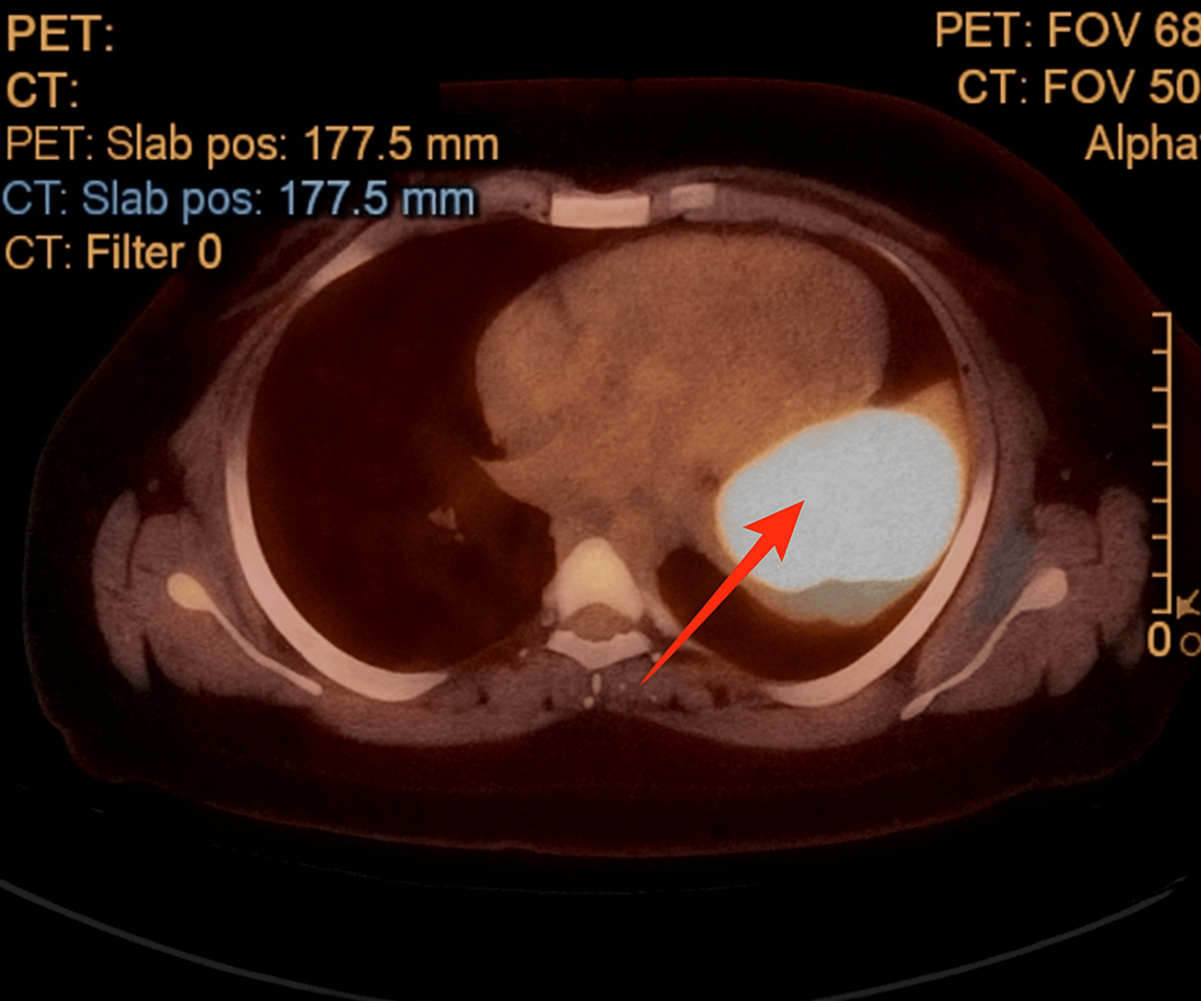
PET/CT scan image performed in April 2023 The image shows a hypermetabolic left upper lobe mass (red arrow). PET/CT: Positron Emission Tomography/Computed Tomography.
